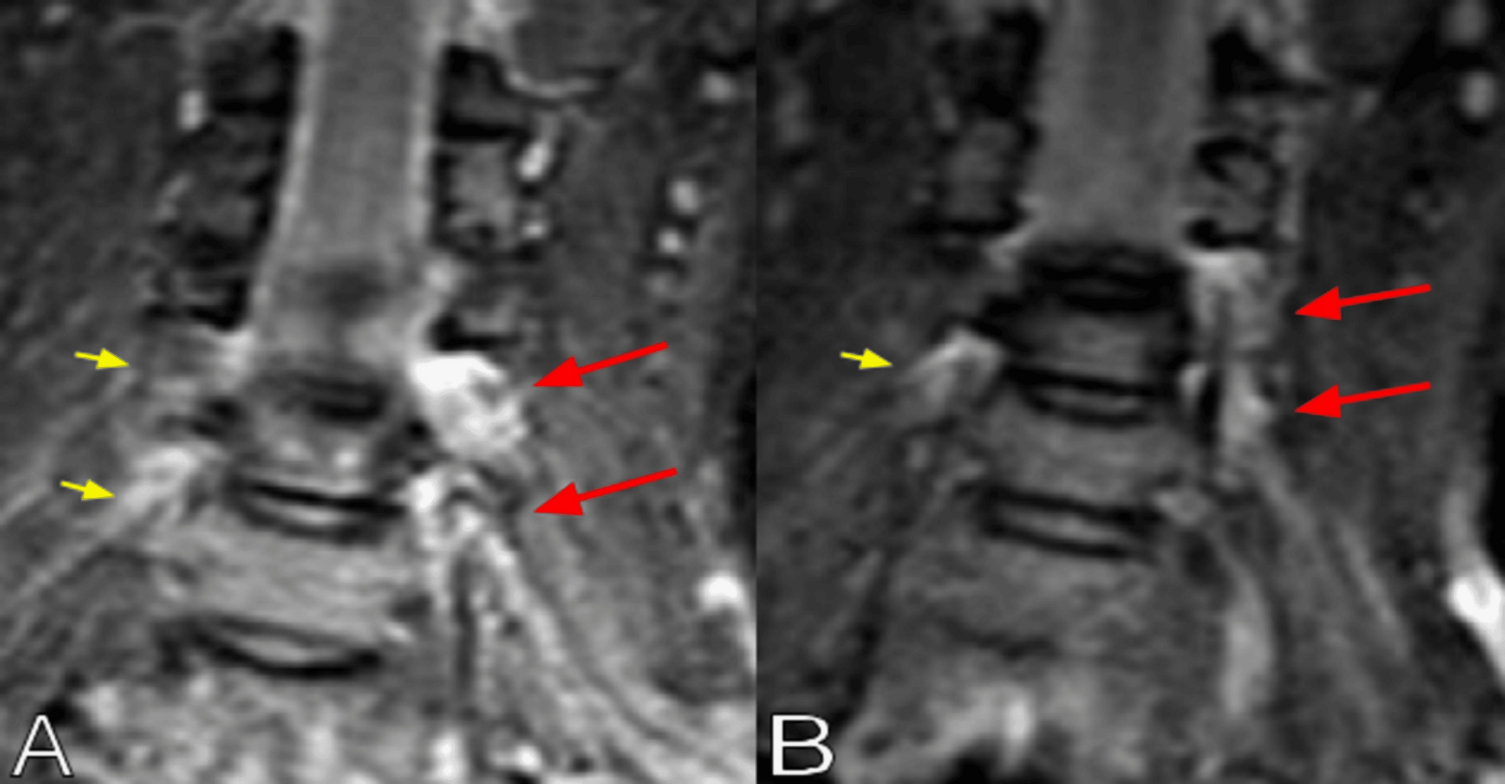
Brachial plexus coronal MRI short tau inversion-recovery (STIR) images showing (A and B) left C4-C5 and C5-C6 nerve root sleeve tears (red arrows), with possible similar contralateral injuries (yellow arrows).
